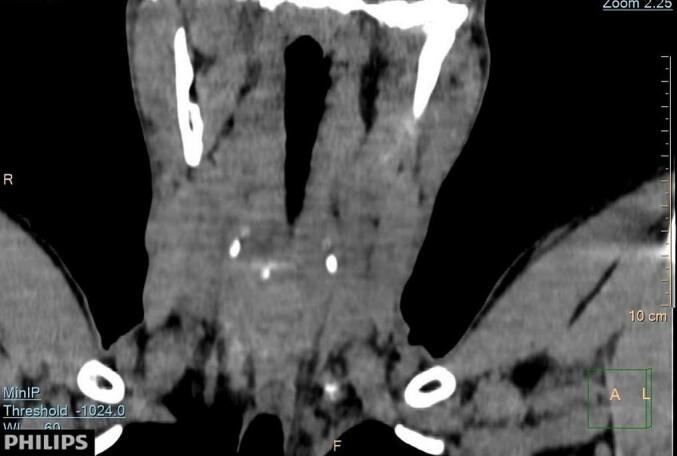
Image of coronal oblique Neck CT shows bilateral moderate ossification of triticeal cartilage.
